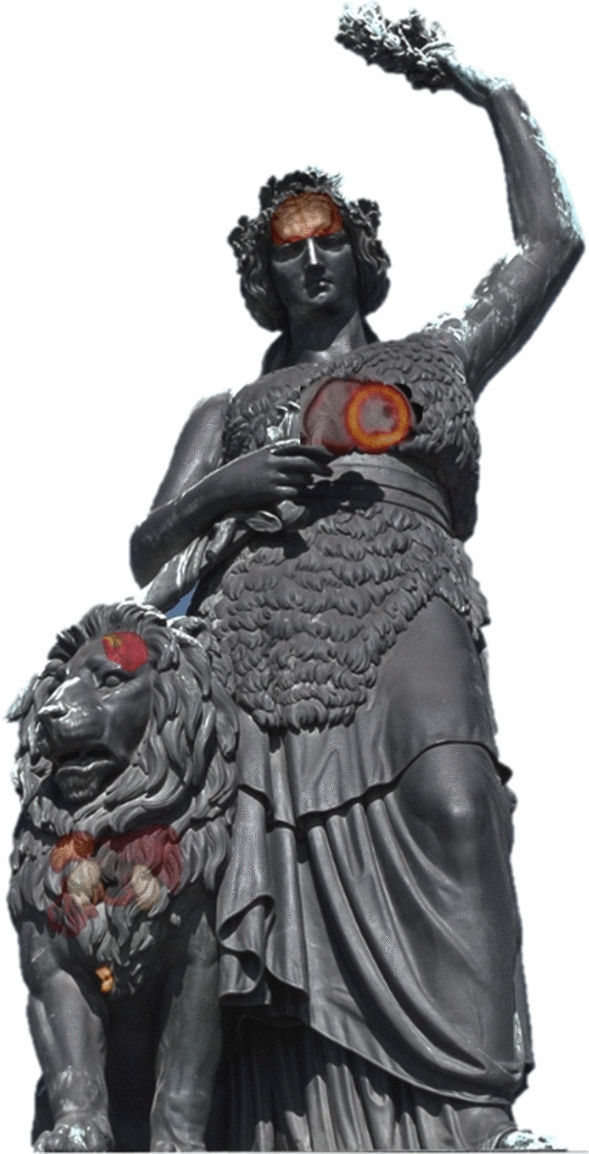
“Bavaria” and her companion adjusted for very particular changes seen in the last decade of PET/MRI
